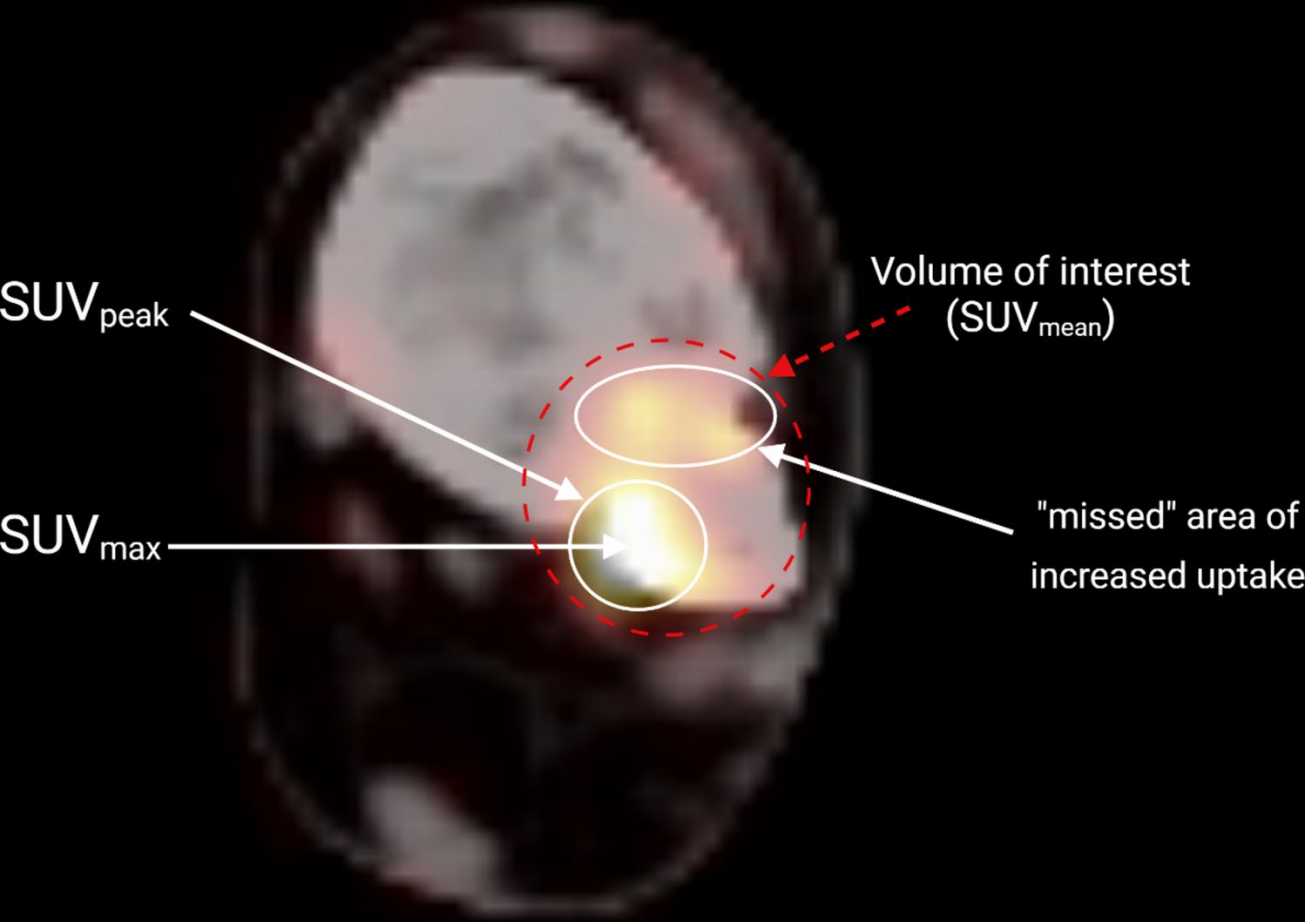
[18F]NaF PET/CT image explaining the difference between volume of interest, SUV_max_, SUV_peak_ and how an area of increased uptake can be “missed” when using SUV_max_ or SUV_peak_
